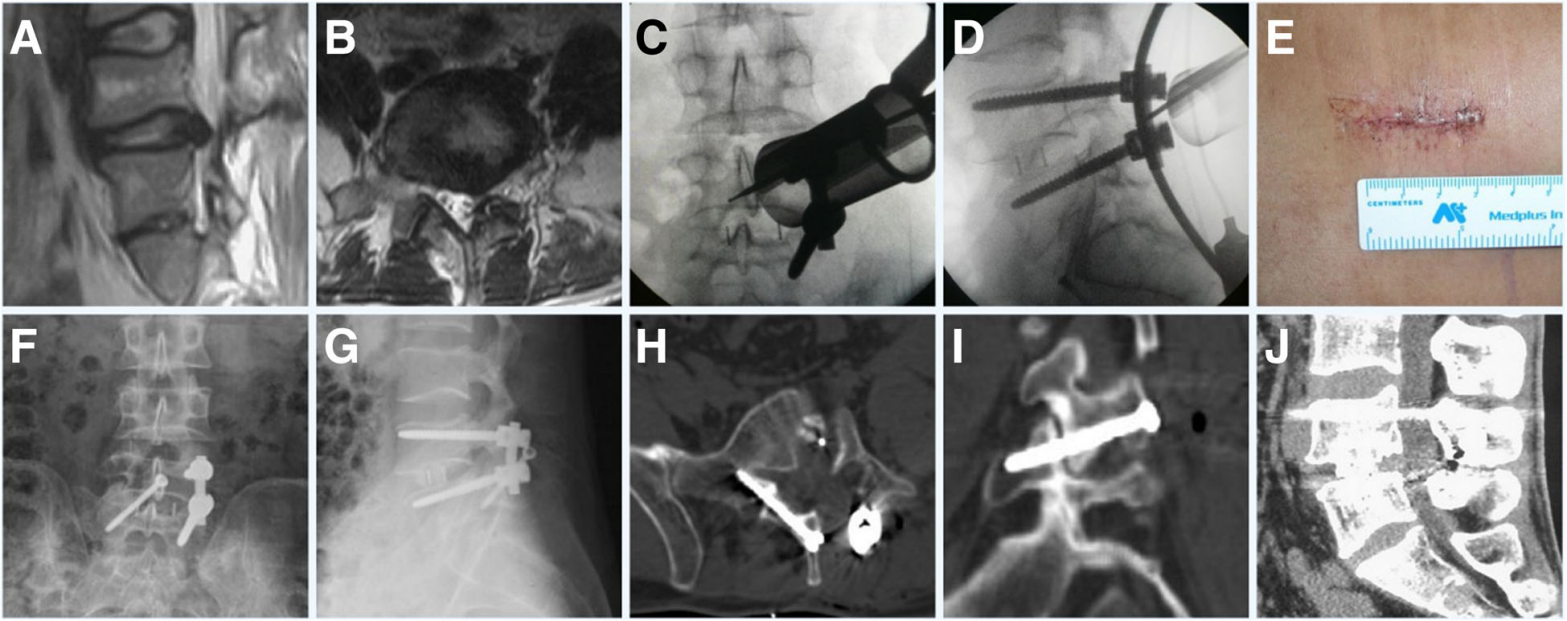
The preoperative MRI (a, b) showed a herniated disc. Accurate placement of the translaminar facet screw (c, d) was accomplished using the C arm intraoperatively. A small skin incision (e) was showed. Anteroposterior (f) and lateral (g) views after MIS TLIF showed good position of the hybrid internal fixation. CT scans (h–j) at 2 years after surgery demonstrated solid interbody fusion, and the translaminar facet screw entered through the base of spinous process on one side, fixed the contralateral facet joint after traversing the laminar, and ended at the base of the transverse process
